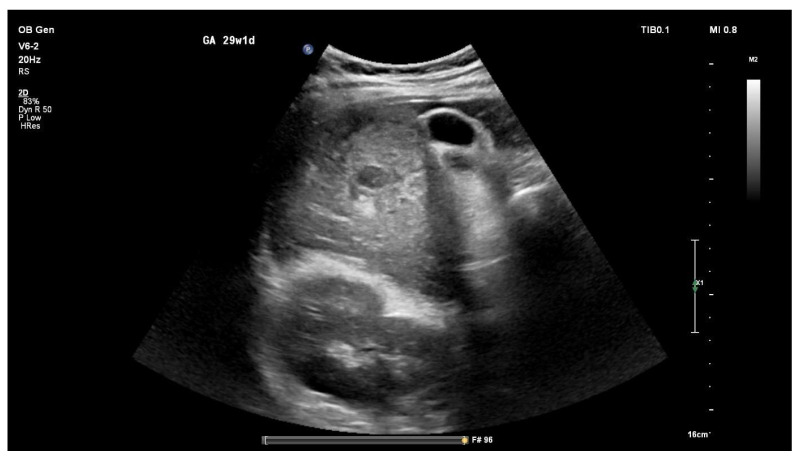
Abdominal ultrasound image demonstrating a cirrhotic liver with irregular, nodular surface contour and coarse, heterogeneous parenchymal echotexture, consistent with advanced chronic liver disease.
